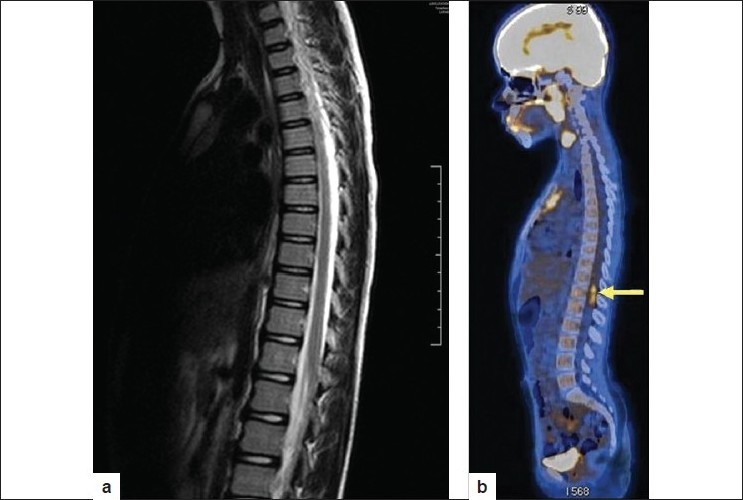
a) Sagittal view MR of Lumbosacral spine showing no abnormal signals; b) Sagittal view FDG PET CT showing linear focus of FDG uptake in D11/12 vertebral level
